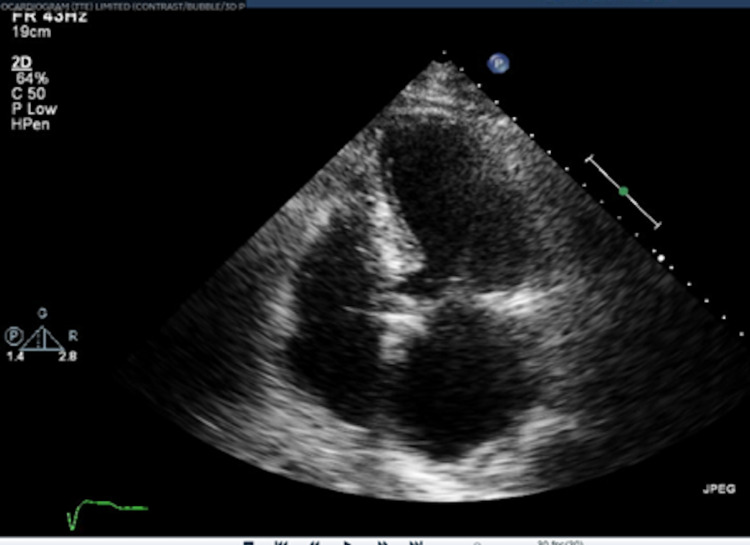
Post-intervention echocardiograph demonstrating improved pulmonary artery pressures and cardiac index of 31/15/17 and 2.3, respectively
